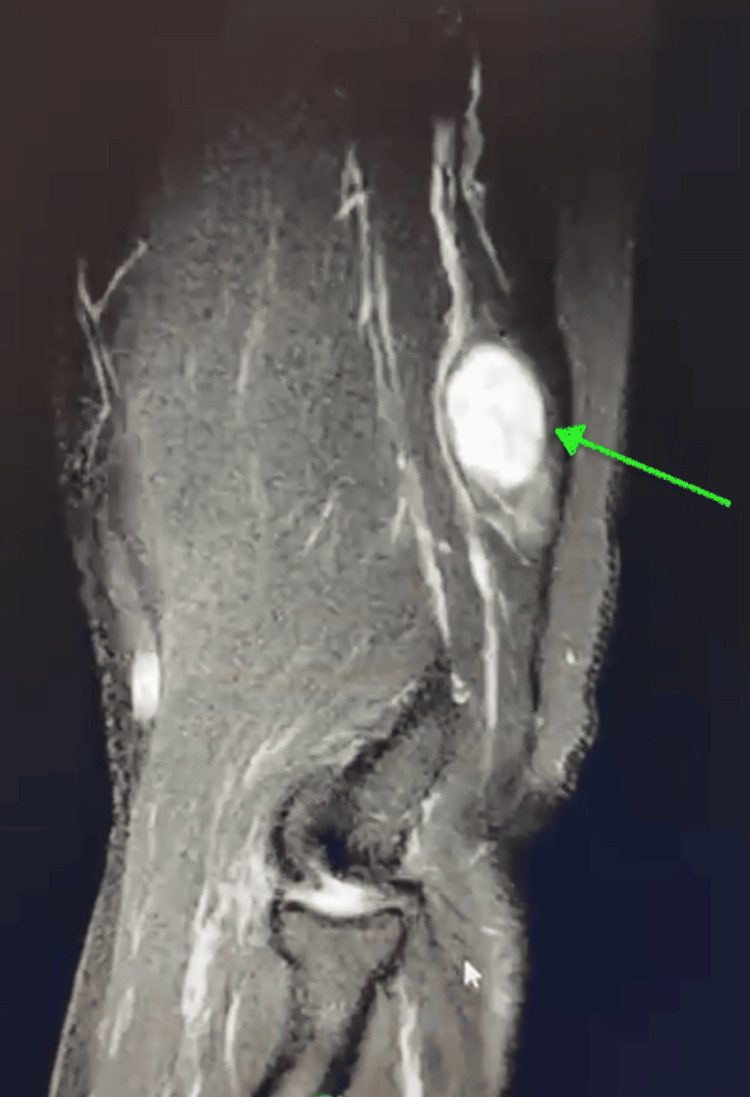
Sagittal view of a contrast-enhanced MRI of the right arm demonstrating a peripheral nerve tumor (green arrow) in the radial nerve
